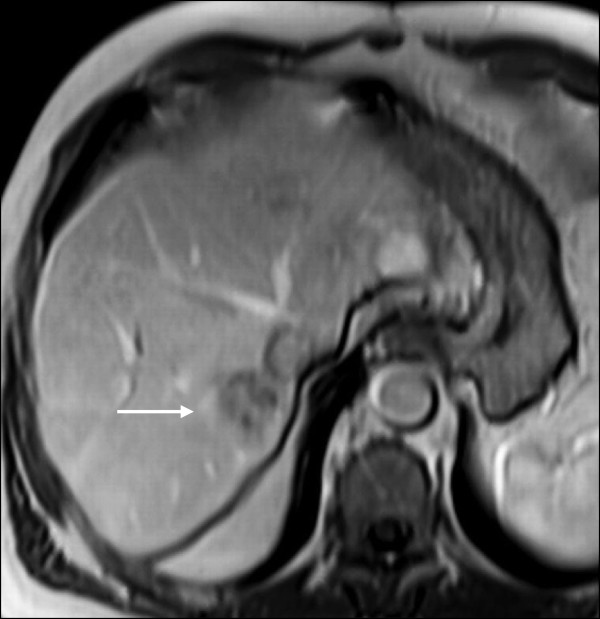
Magnetic resonance imaging of the transplant liver before selective internal radiation therapy (SIRT). The arrow points to the ICC in segment VII of the transplant liver.
